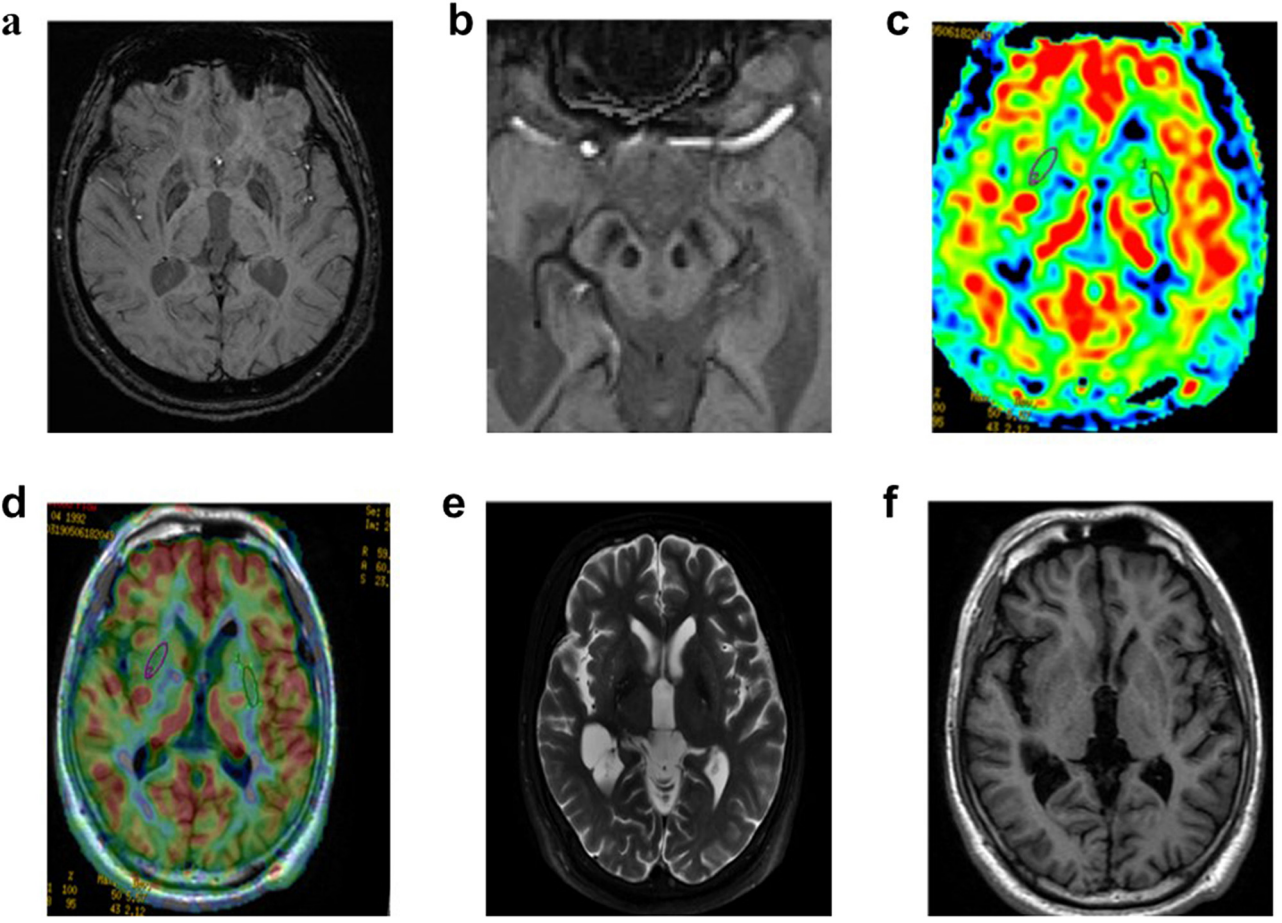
SWI and ASL-MRI of WD. (a and b) SWI showed decreased signal intensities in putamen, globus pallidus, substantia nigra, and red nucleus. (c and d) ASL-MRI demonstrated the reduction of cerebral blood flow in the bilateral putamen. (e) T1WI, and (f) T2WI showed substantially normal in the putamen, globus pallidus, substantial nigra, and red nucleus.
